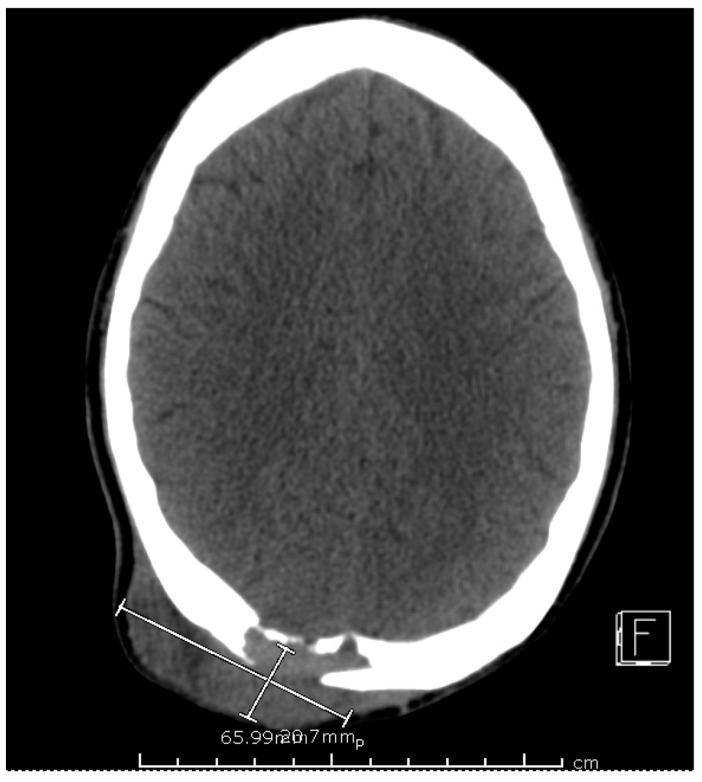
CT scan of head, aggressive lytic lesion of the posterior right parietal bone with an associated 6.6 × 2.1 × 7.1 cm soft tissue mass of the posterior scalp.
